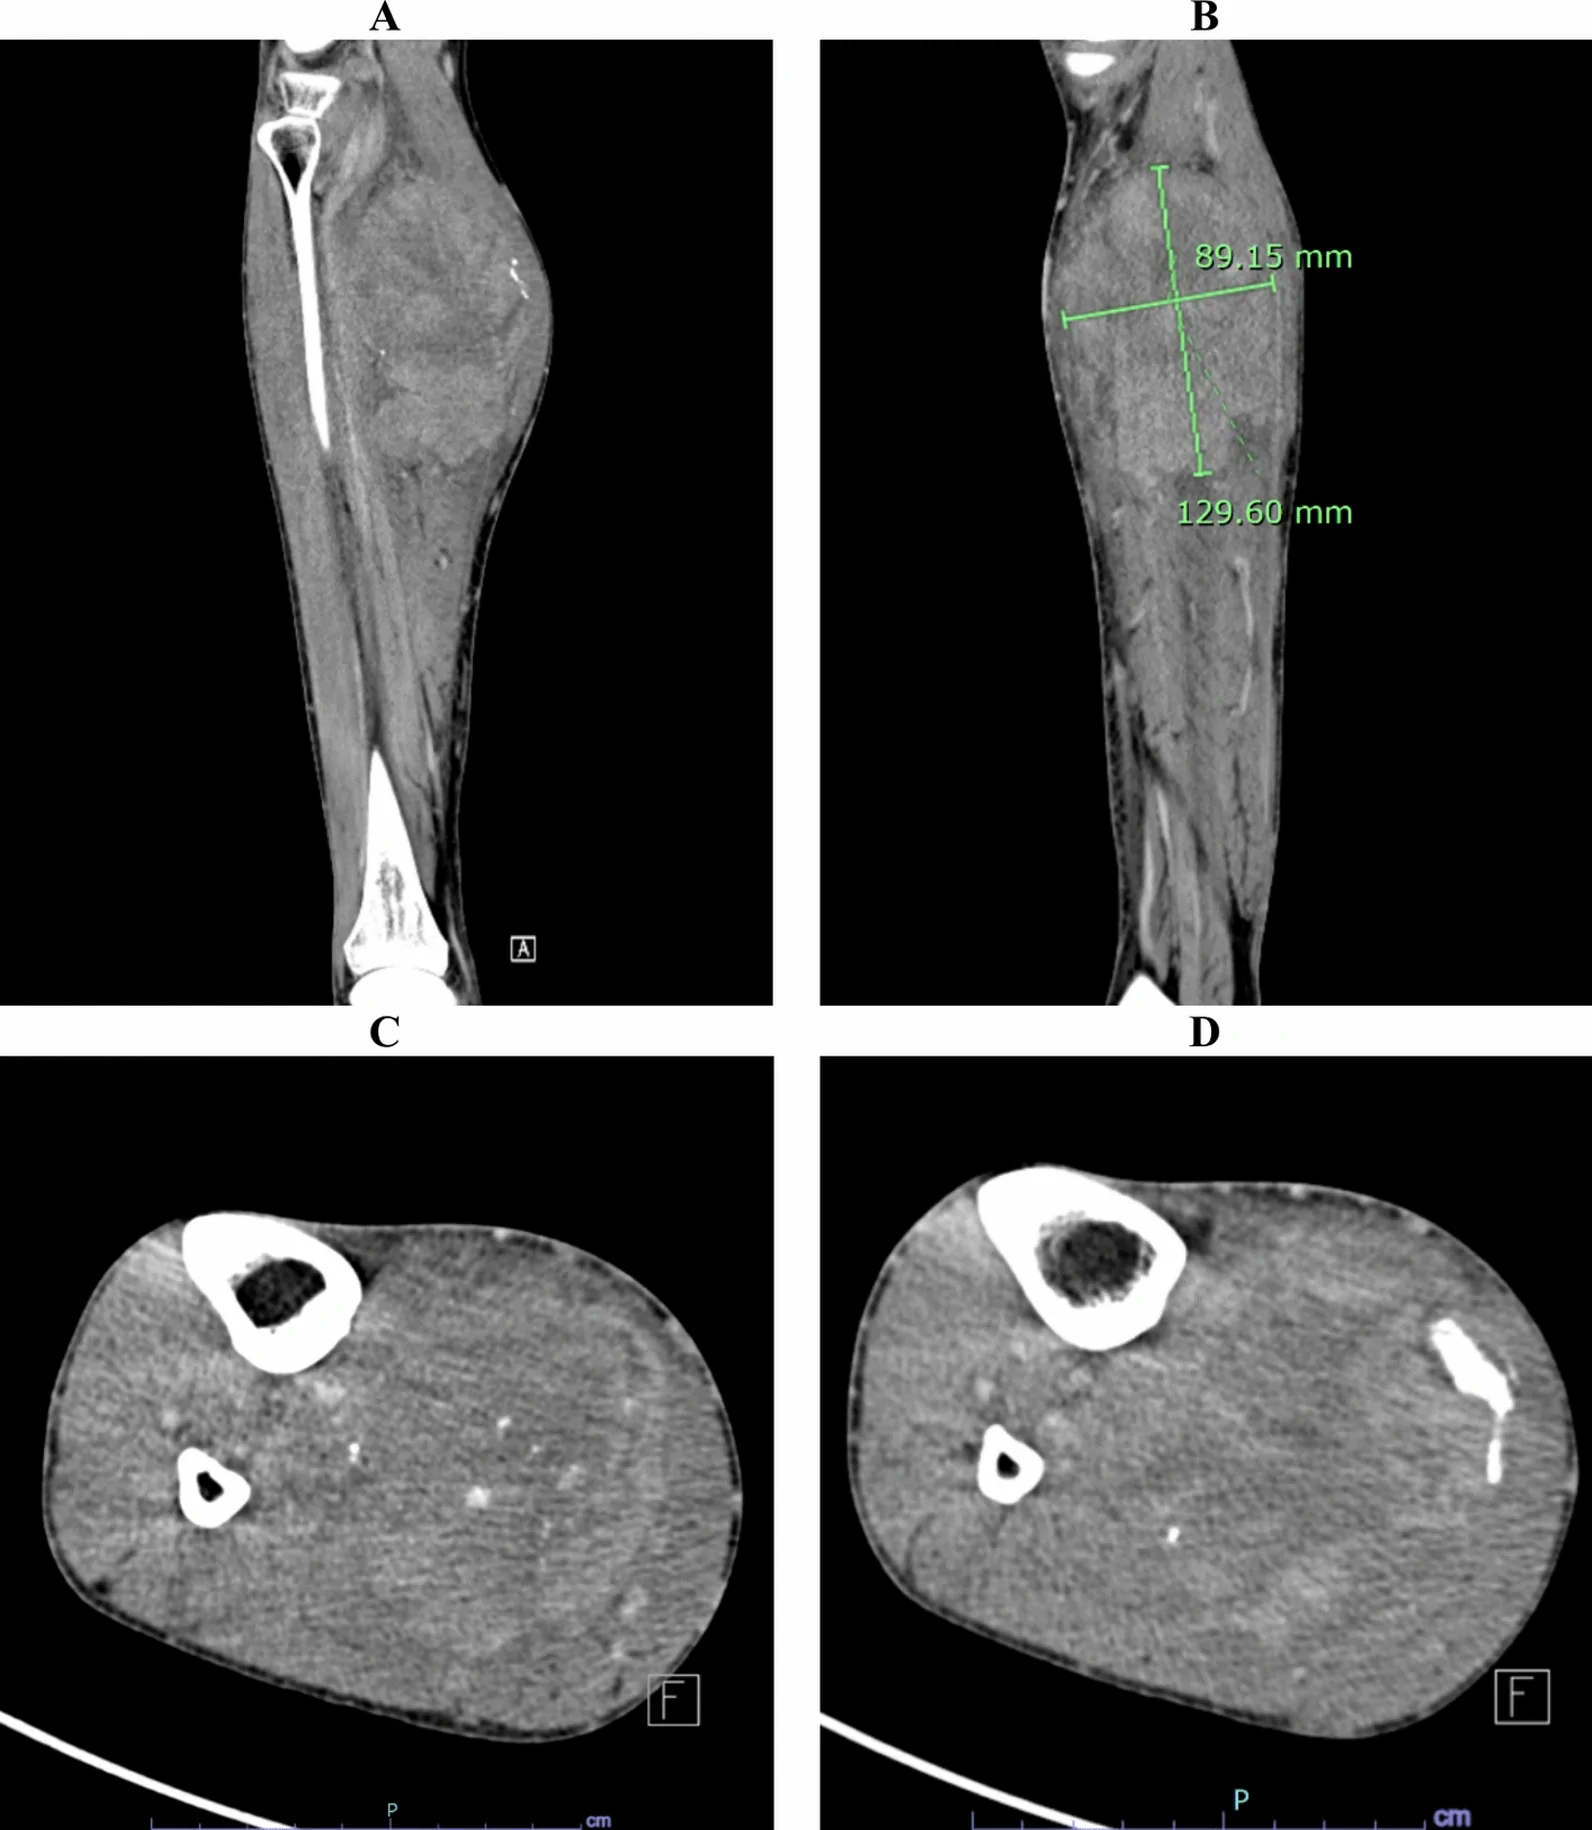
CT of the right tibia and fibula obtained on May 14th, 2024, showing an 8.9 × 8.7 × 13.0 cm lobulated and heterogeneous soleus intramuscular mass with multiple peripheral and internal calcifications in coronal (A), sagittal (B), and two axial views (C) and (D)
